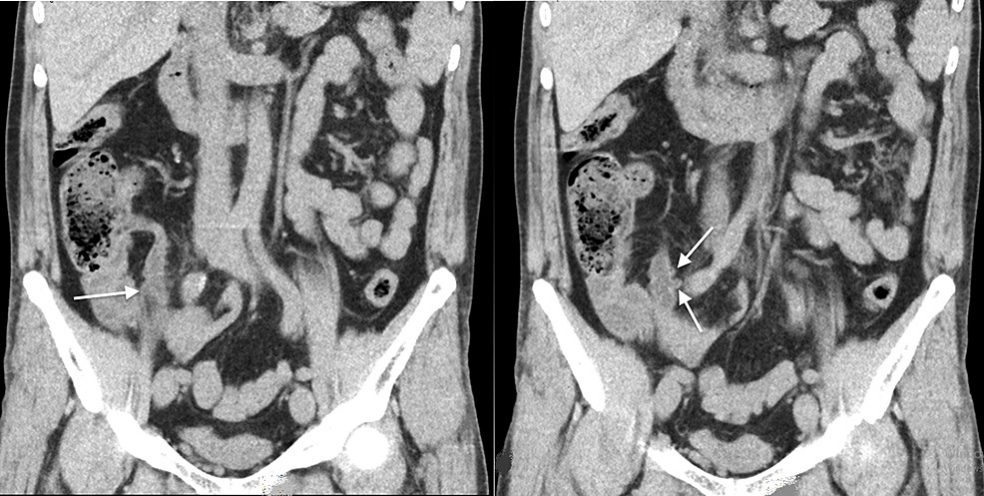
Coronal images of the abdominal computed tomography scan showing multiple diverticula of the appendix (white arrows).
